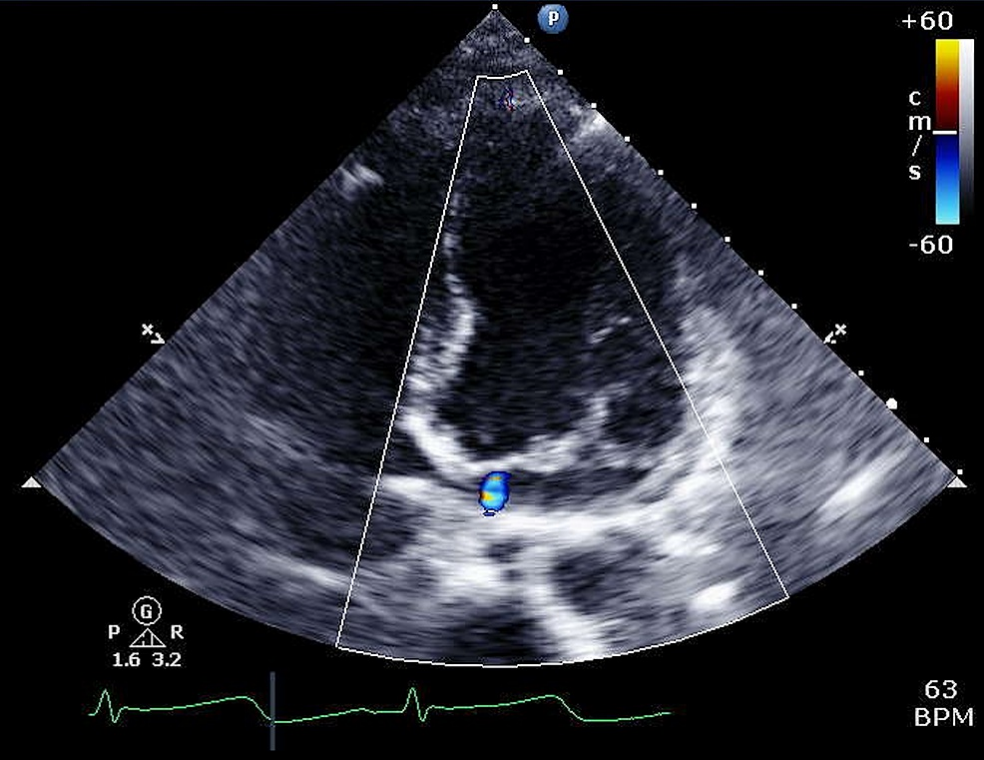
Initial echocardiogram showing four-chamber view with color Doppler
